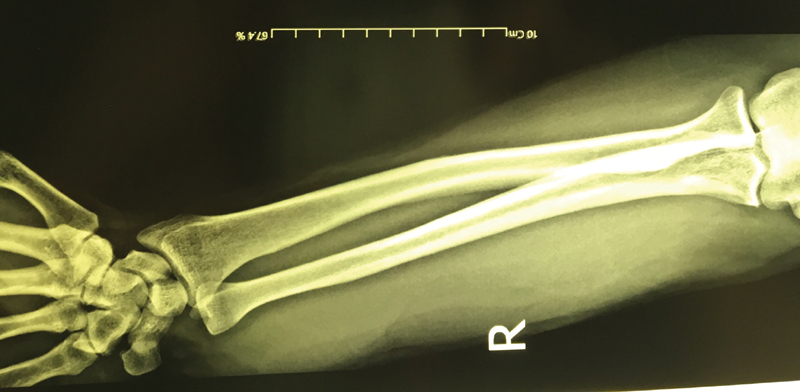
Conventional radiography of the right wrist and forearm showing a soft tissue mass and swelling in distal one-third of the forearm without bone involvement.
